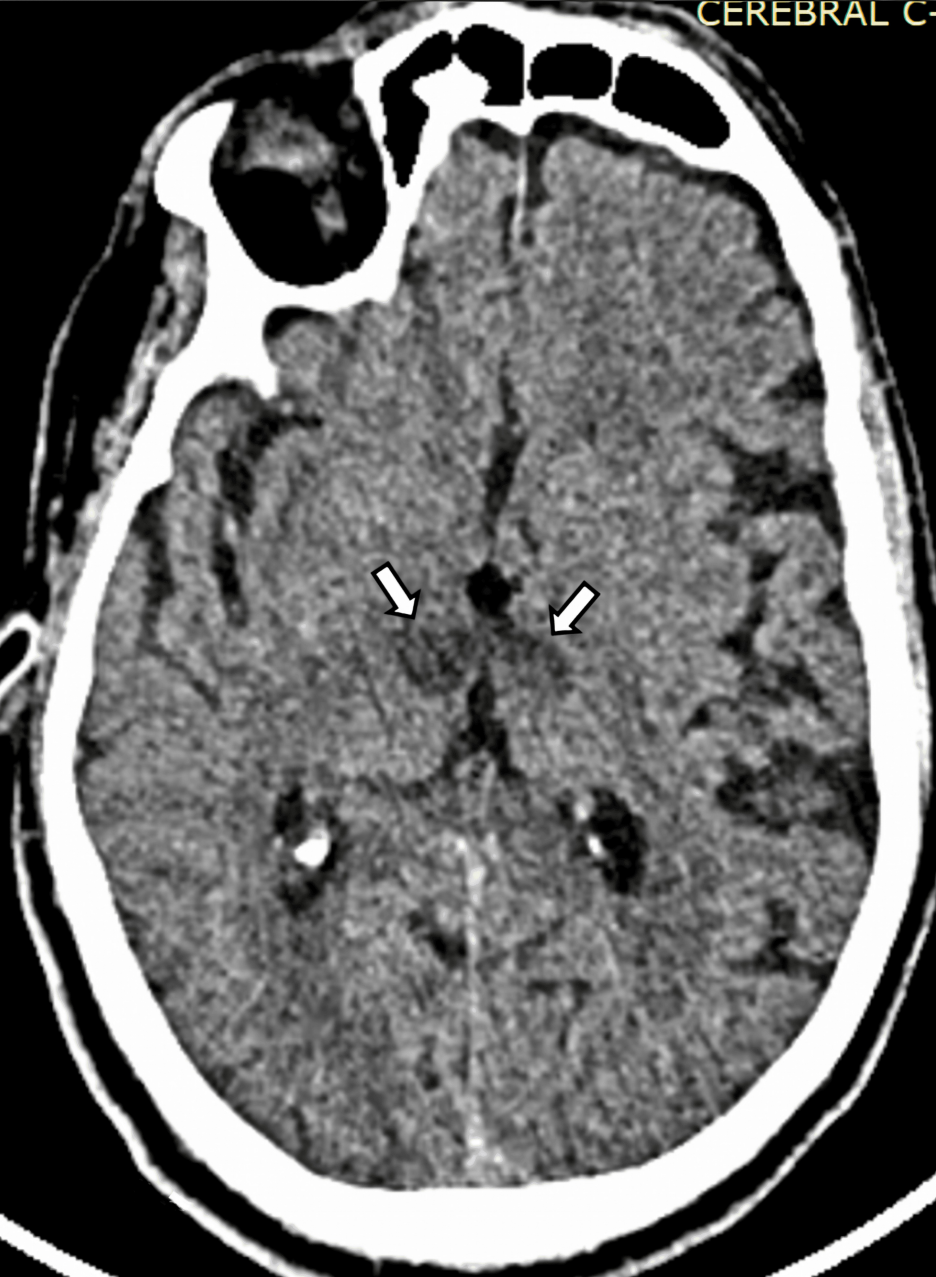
Non-enhanced CT performed 24 hours after IVT revealed bilateral hypodensities in the paramedian thalamic regions (white arrows) without hemorrhagic transformation, confirming the diagnosis of an AOP infarction. AOP: artery of Percheron; IVT: intravenous thrombolysis
